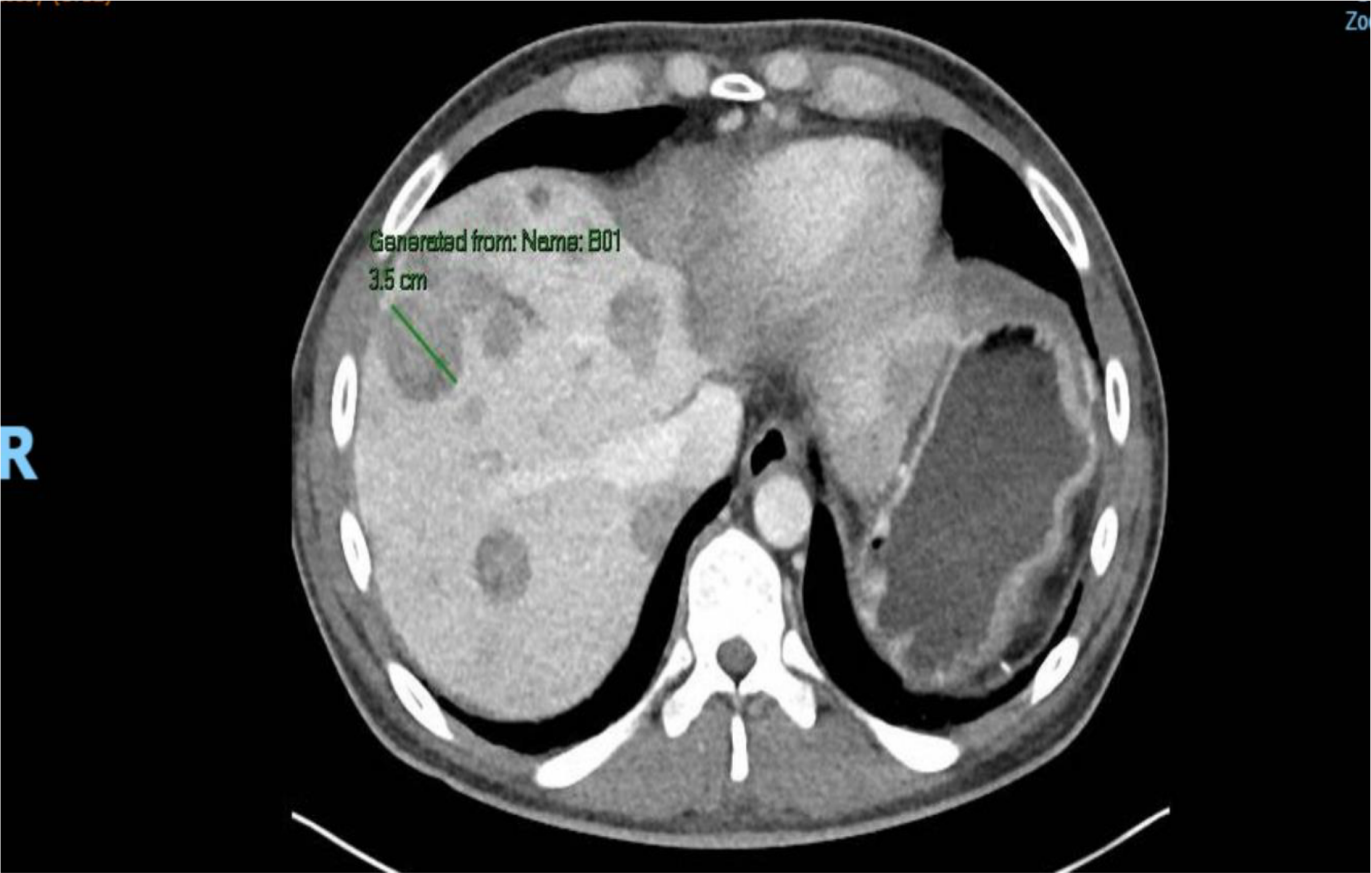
CT A/P post-debulking of mass and initiation of imatinib. Imaging showed notable improvement in hepatic metastasis in size and number.
